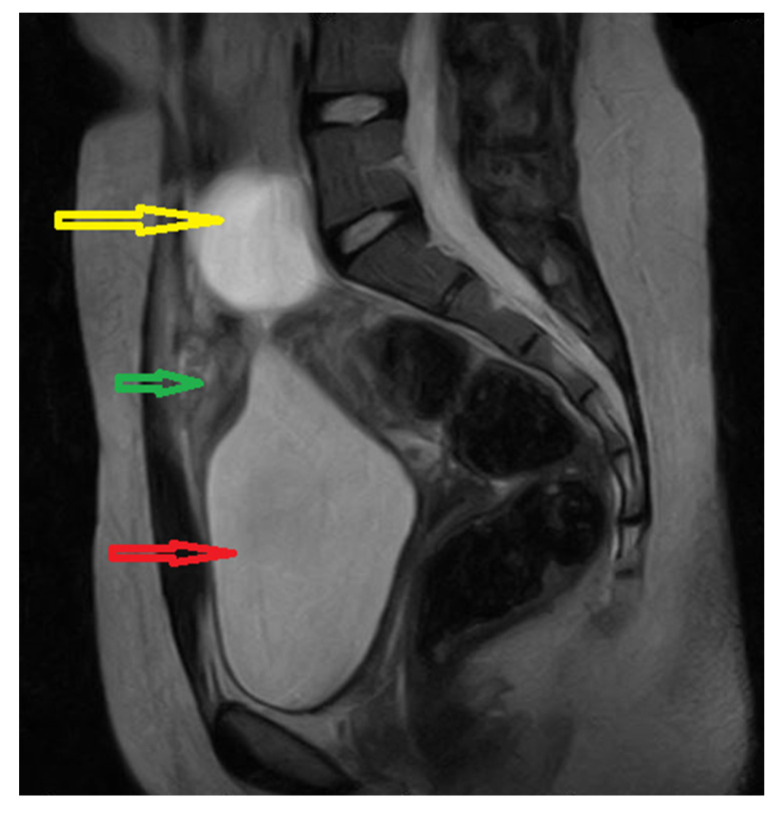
Pelvic MRI image: Sagittal T2-weighted yellow arrow: left ovarian cyst; green arrow: supposed urachal cyst; red arrow: urinary bladder.
